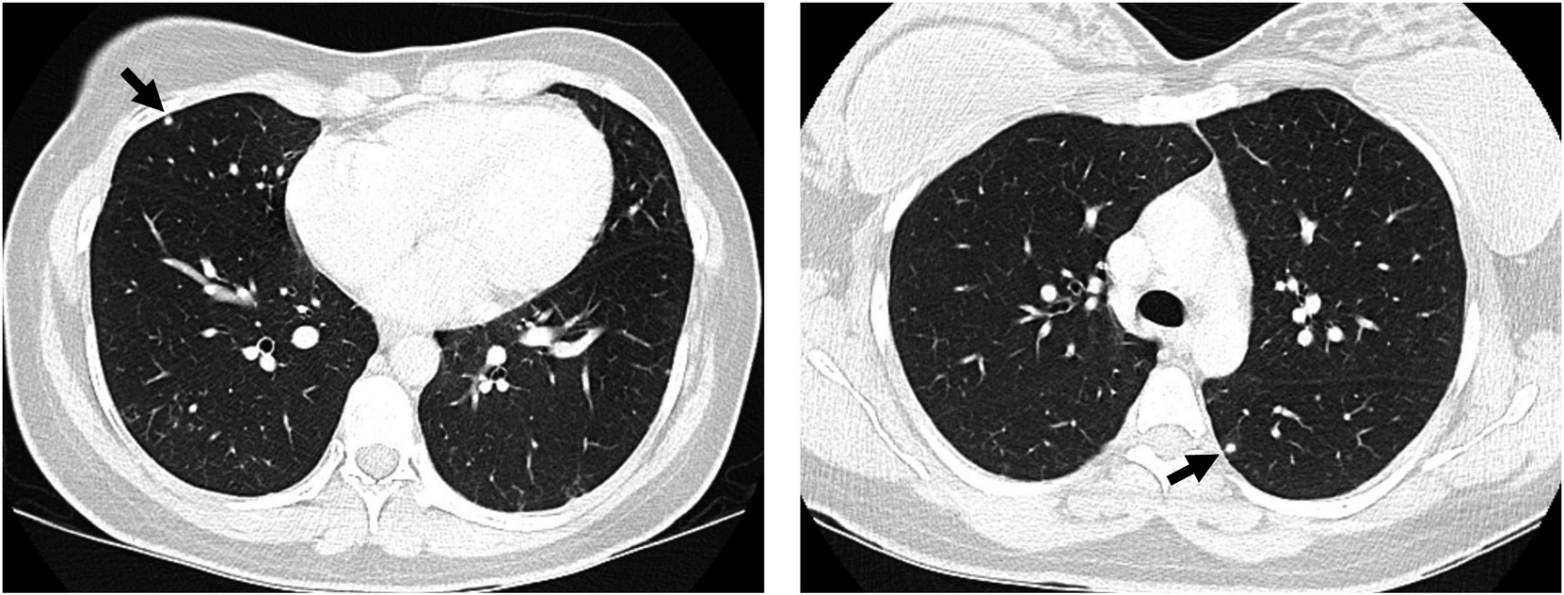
Tumor staging. Computed tomography (CT) scan revealed several small bilateral pulmonary metastases (arrows).
